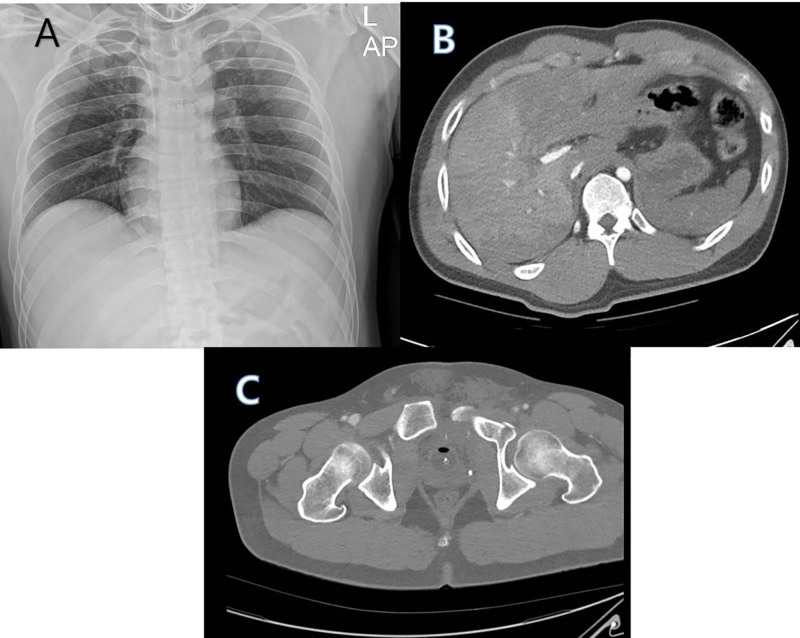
Simple chest radiography (A) shows no specific findings. Abdominal computed tomography (B and C) showing pelvic and sacral fractures, a hematoma in the pre-vesical space, and liver contusion.
